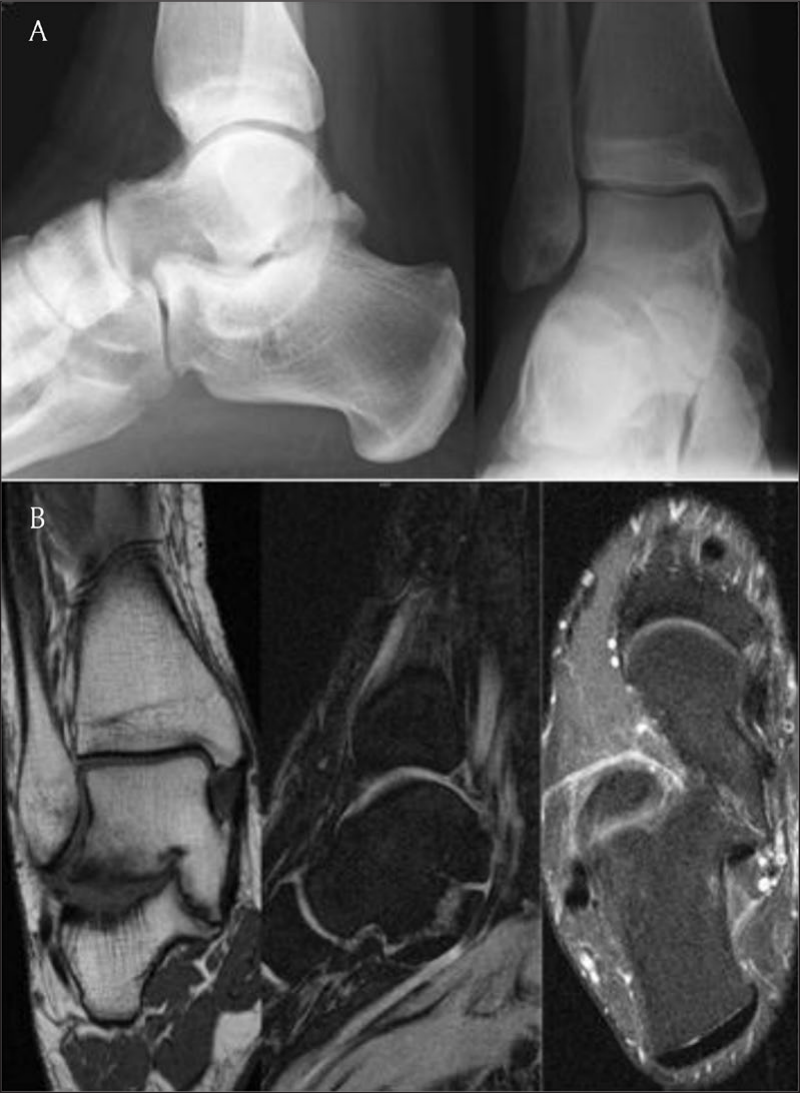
A 23-year-old male patient complains about obtuse pain in the right hindfoot initiating spontaneously, without a history of trauma and increasing under loading since one year. (a) Standard radiographs show no evidence of OA, inflammatory disease or bone destruction. But one can appreciate a C-sign (i.e. there is an apparent continuity in the bony contour of the talar dome to the sustentaculum tali) and the sustentaculum tali does not show the typical ‘brick’ shape but appears somehow roundish in the lateral view. Note the small bony prominence somewhat posterior to the margin of the talonavicular joint, consistent with a subtle talar beaking. In the frontal view, note the abnormal angulation of the middle talocalcaneal joint facet from the coronal towards the sagittal plane. (b) MRI (from left to right: T1w cor, Flash 3D Water Excitation sag, moderately T2w FS ax) reveals a fibrocartilaginous coalition of the middle talocalcaneal joint facet and demonstrates the abnormal angulation of the joint facet to more detail. There is but subtle periarticular sclerosis and bone marrow edema pattern.
